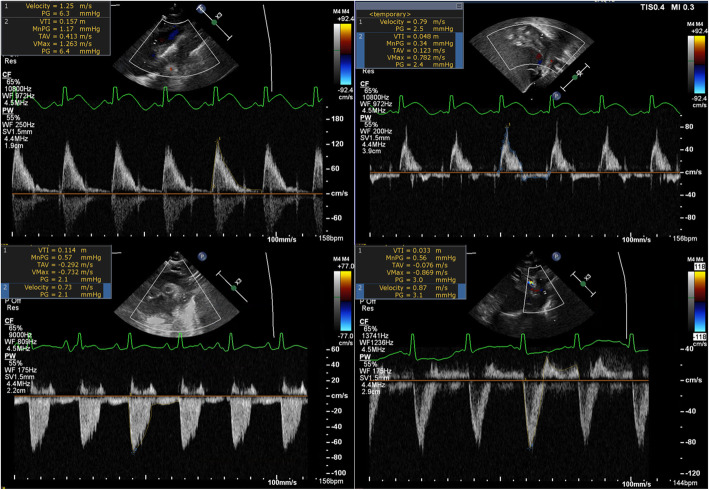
Pulse-wave Doppler envelopes in the Aorta. Legend: Peak systolic velocity and velocity time integral of the spectral envelope obtained by pulse-wave Doppler at various level in the Aorta. Panel a: Ascending aorta in the suprasternal view; b: Descending Aorta in the subcostal view (retrograde flow in diastole); c Post-ductal descending aorta in the suprasternal view (anterograde flow in diastole); d Post-ductal descending aorta in the suprasternal view (retrograde flow in diastole)
